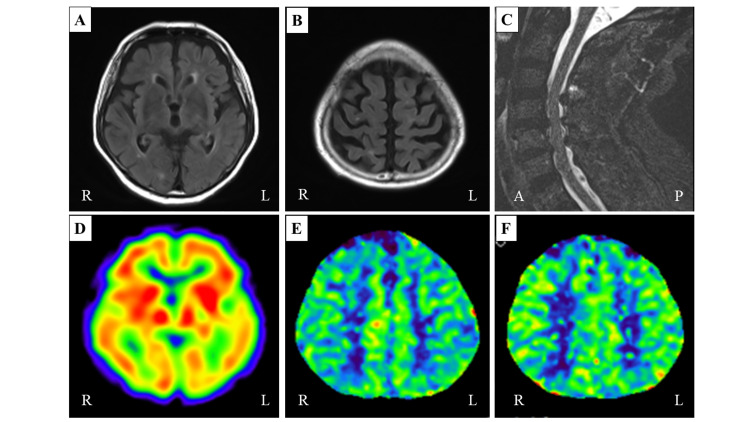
Clinical examinations of the patient. (A, B) Brain MRI showing no abnormality. (C) Spinal MRI showing mild cervical spondylosis. (D) IMP-SPECT showing no blood flow decrease in the brain cortex. (E-F) There is no change in cerebral blood flow on the perfusion CT between pre-dialysis (E) and post-dialysis (F). IMP: N-isopropyl[123I]-p-iodoamphetamine; SPECT: Single photon emission computed tomography.
